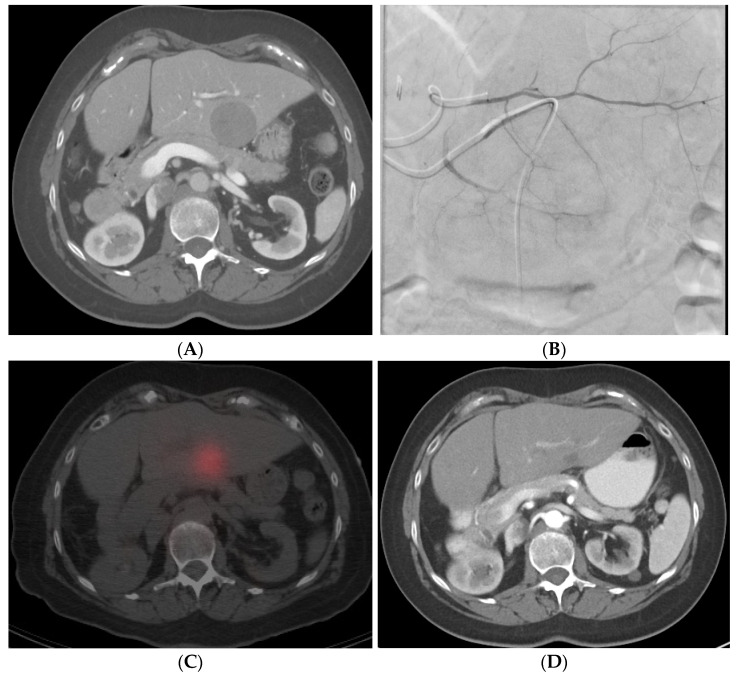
Radiation segmentectomy in a patient with HCC with a past surgical history of right hepatectomy. (A) CT showing a large lesion in the left lobe of the liver. (B) Left hepatic artery angiogram showing vessels supplying the tumor. (C) SPECT-CT imaging after left segment-2 sub-segmentectomy, showing good dose delivery. (D) Follow-up CT showing good response in the treated area.
